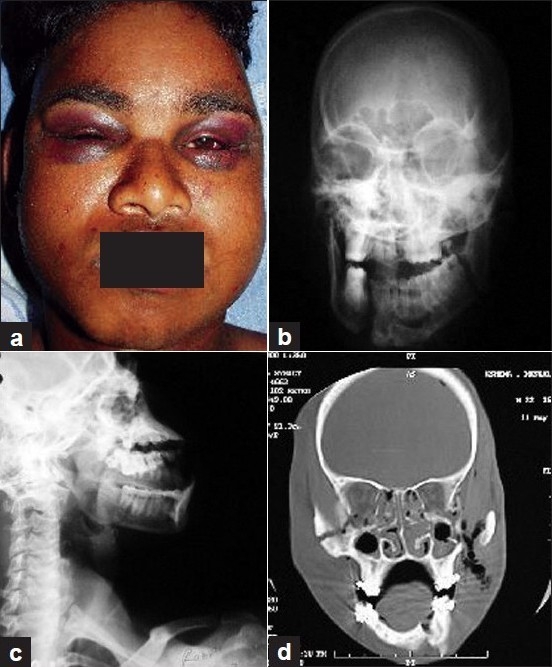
(a) Pre-operative images showing bilateral black eyes in a patient with craniofacial trauma. (b) X-ray skull: AP view of the same patient showing multiple fractures of facial bone including mandible. (c) X-ray skull: lateral view of the same patient showing multiple fractures of facial bone including mandible. (d) CT scan of the same patient showing details of facial bone fractures. Patient was treated surgically and internal fixation of bone fragments was performed
